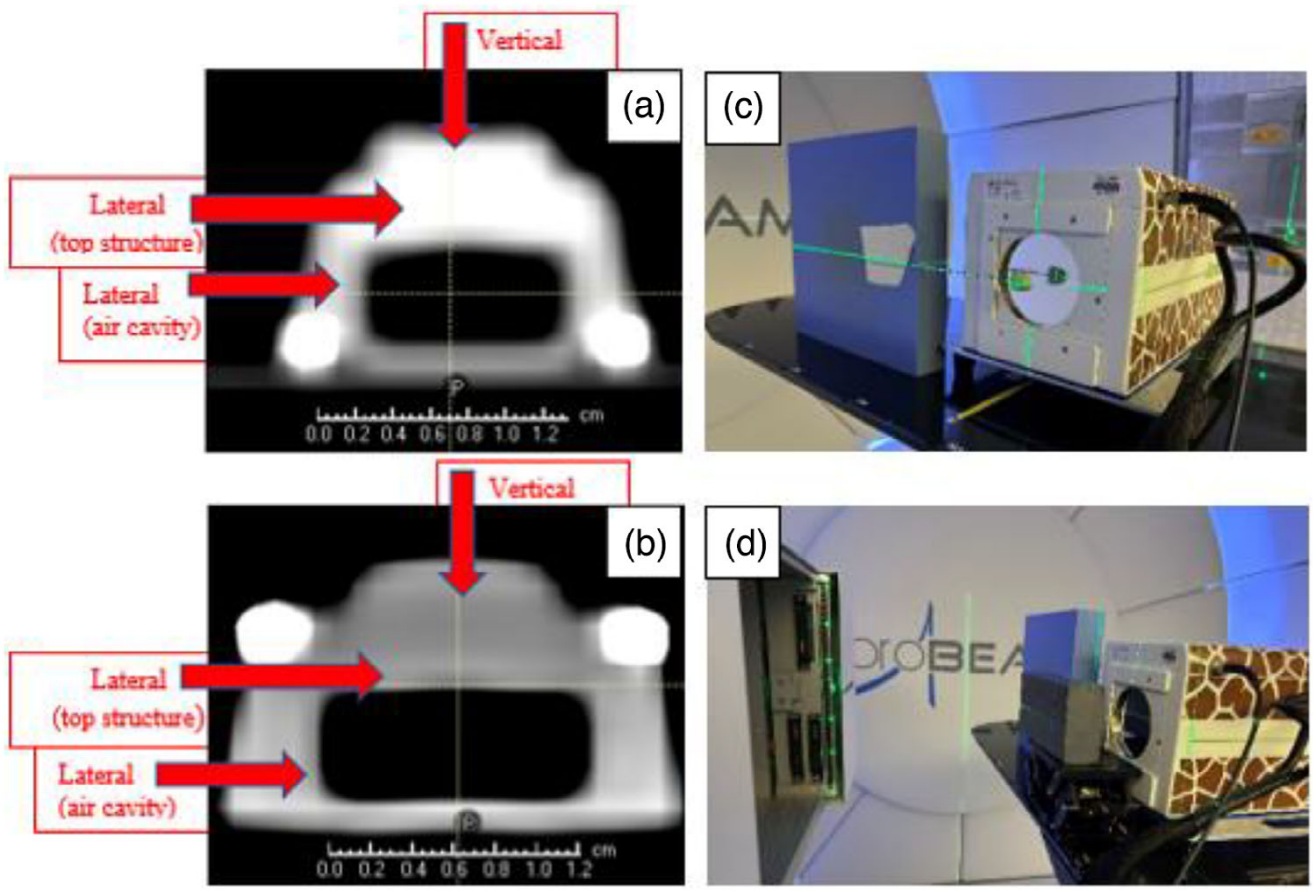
CT image of two types of chemo‐ports and the measurement setup for acquiring the integrated depth doses (IDDs) with and without a collimator. A stick attached to the stem of the chemo‐port is used to adjust the orientation of the chemo‐port easily. The red arrows indicate the beamlet directions, vertical and lateral to the chemo‐port. (a) slim port, (b) isp port, (c) setup without a collimator, and (d) setup with a collimator.
